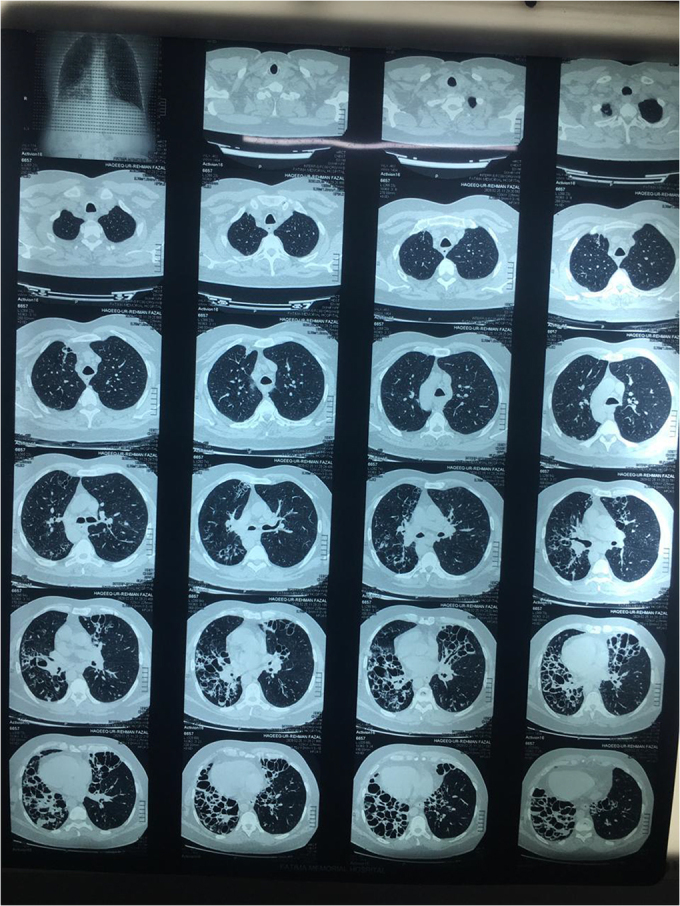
HRCT chest and abdomen showing dextrocardia, left-sided liver, and right-sided spleen, thus indicating situs inversus.
